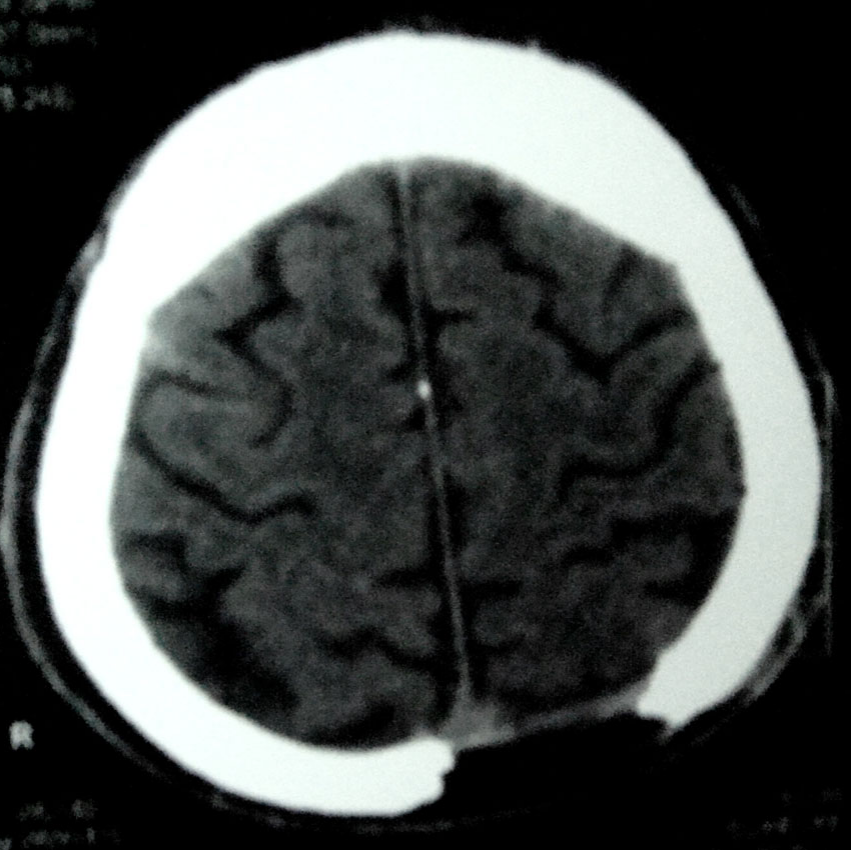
Computed tomography of the skull. The tumor appears as a lytic area with sharp borders. Both of brain hemispheres seem intact.
